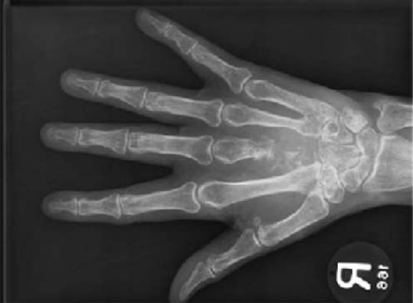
Metastatic breast carcinoma in the third metacarpal and base of the fifth metacarpal. Radiograph of the right hand reveals extensive lytic destruction of the proximal two thirds of the middle metacarpal plus permeative lytic change in the base of the fifth metacarpal.
